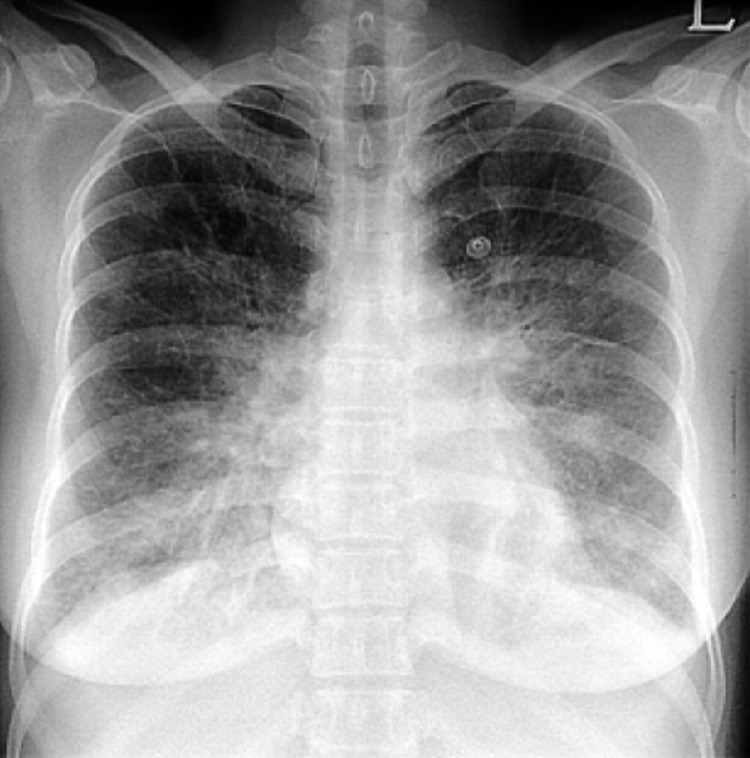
Chest X-ray showed pulmonary edema
